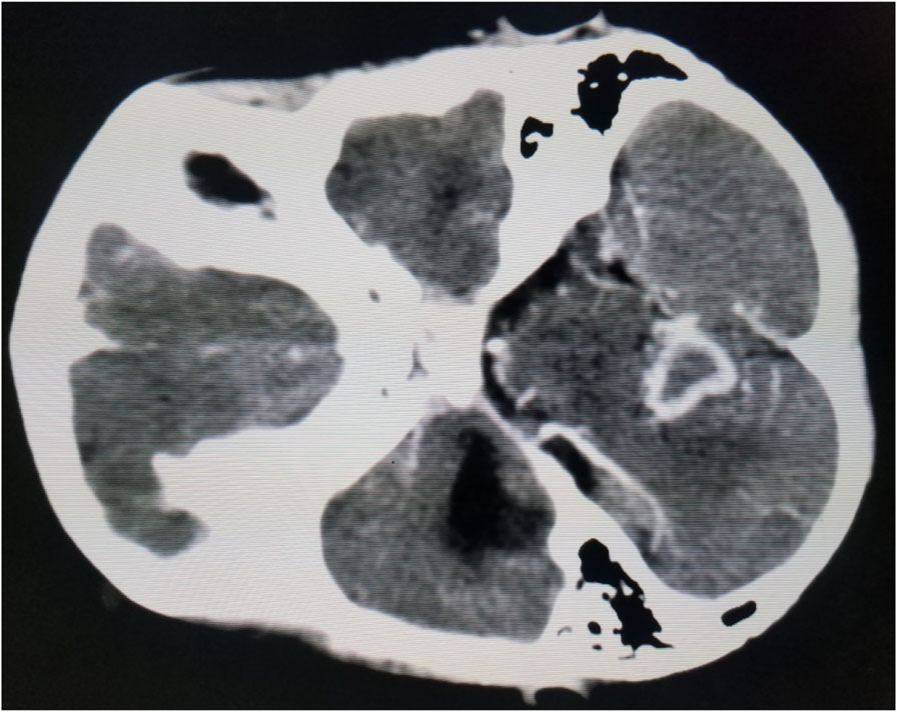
Brain computed tomographic scan showing ring enhancing lesion with perilesional edema
